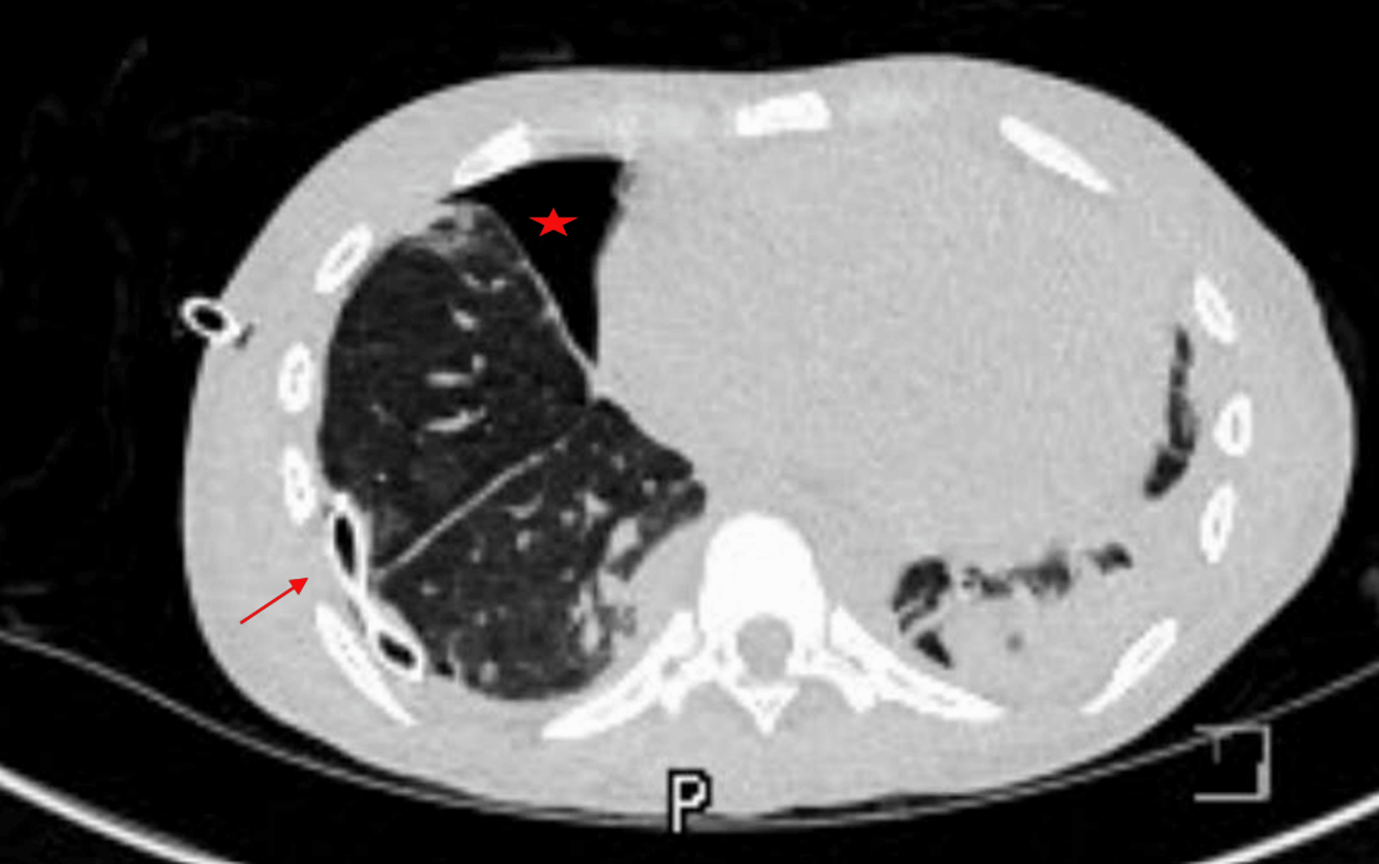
CT chest: enlarged heart, pneumothorax (red star) with chest tube (red arrow) in place CT: computed tomography
